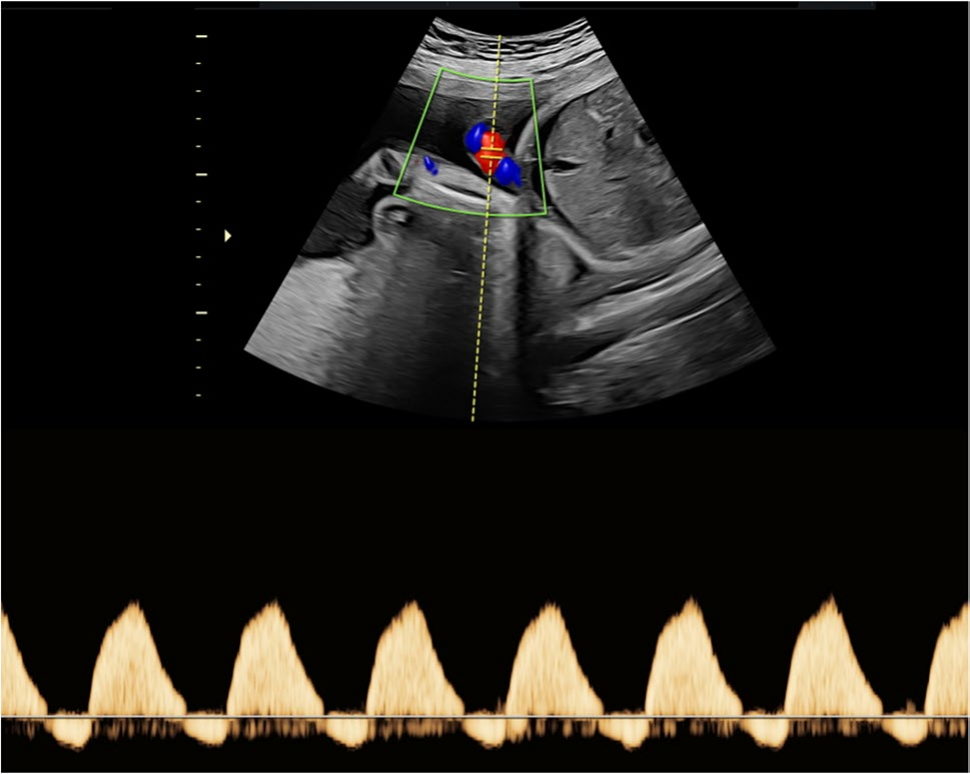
Case 2 at 31 + 6 weeks of gestation, CVPS 4 points (ascites, pleural effusions, generalized skin edema,: 0 points, mild cardiomegaly (cardiothoracic area ratio = 0.45): 1 point, competent tricuspid and mitral valve: 2 points, umbilical artery Doppler velocimetry with reverse end-diastolic flow: 0 points, ductus venosus with reversed a wave: 1 point)
